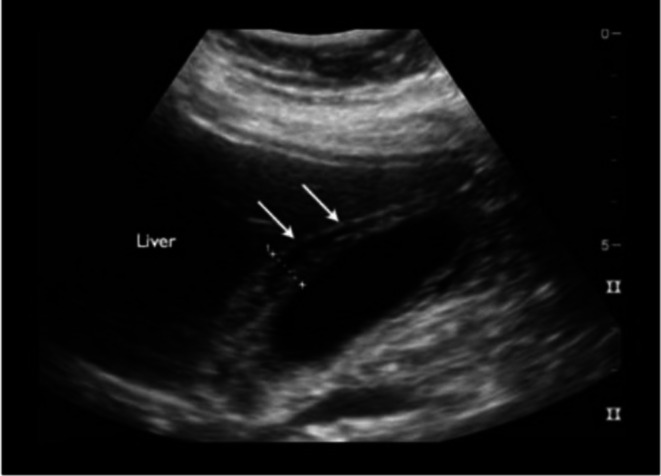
Longitudinal ultrasound view of the gallbladder demonstrating circumferential thickening of the anterior wall with mild pericholecystic fluid collection, indicative of acute acalculus cholecystitis (white arrows).
